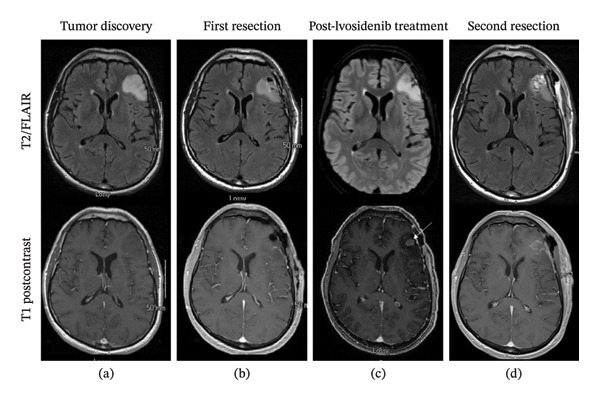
MRI brain imaging. Axial T2/FLAIR and postgadolinium T1‐weighted sequences, depicting the tumor at four time points: (a) initial discovery, (b) following subtotal resection performed 2 months later, (c) after 4 months of ivosidenib treatment with enhancement noted by white arrow in T1 postcontrast imaging, and (d) following second resection 1 month later (5 months after initiation of ivosidenib treatment).
